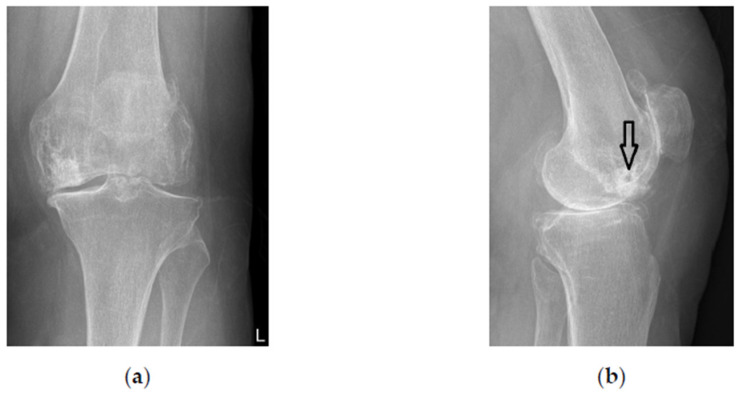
Standing X-rays of the left knee 4 years after subchondroplasty. Note calcium phosphate compound (CPC) filling the subchondral bone of the medial femoral condyle. (a) Anteroposterior view; (b) lateral view. The hole from the delivering cannula is clearly visible (arrow).
